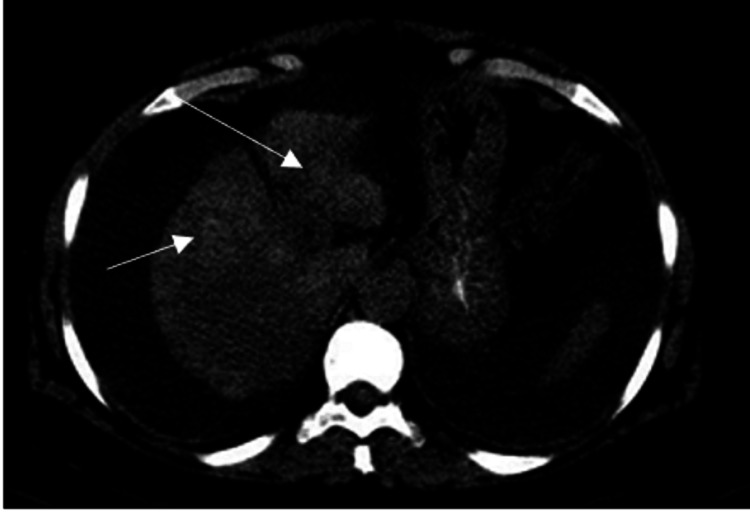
CT scan showing hepatic atrophy
